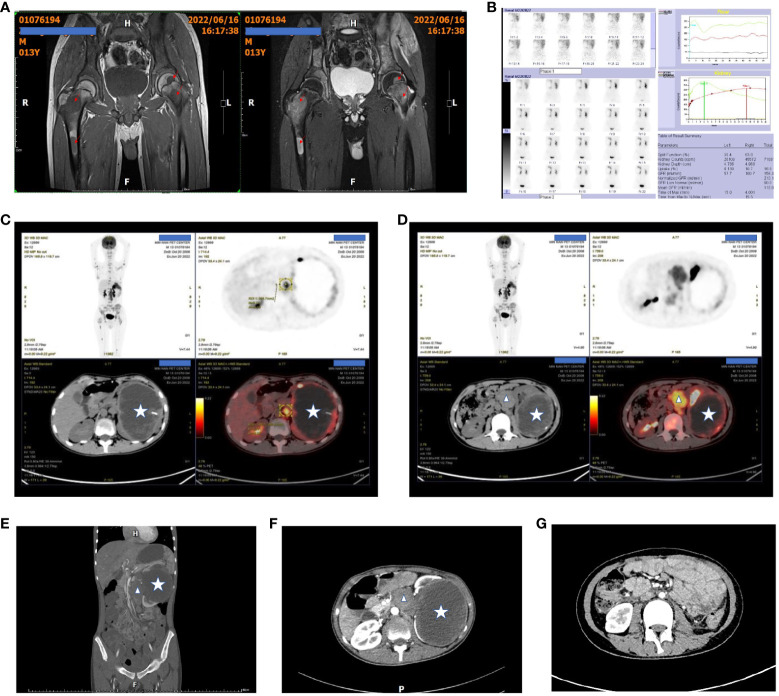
Patient’s pre- and post-treatment imaging data. (A) Preoperative hip MRI shows multiple tumors in both femora, with prolonged T1 and T2 signals and high T2-weighted fat-suppressed signals. The arrows indicate the tumor lesions. (B) Dynamic renal imaging shows a large, hypovascular mass in the left kidney. (C, D) PET-CT scans show a left renal malignant tumor with multiple distant metastases. The star indicates the kidney tumor, and the triangles indicate the metastasis to the inferior vena cava and abdominal aorta. (E, F) Preoperative abdominal contrast-enhanced CT scans show the left renal tumor and the metastasis to the inferior vena cava. (G) Abdominal contrast-enhanced CT scans at 6 months after the second-stage surgery show no recurrence of intra-abdominal tumors.
